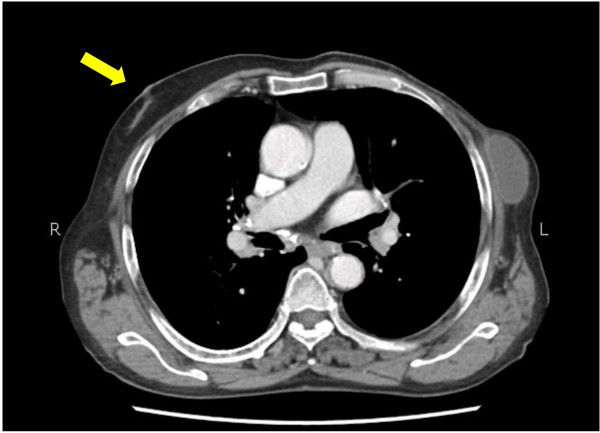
Computed tomographic image highlighting right sided gynaecomastia (yellow arrow) and left sided postoperative changes with seroma formation.
